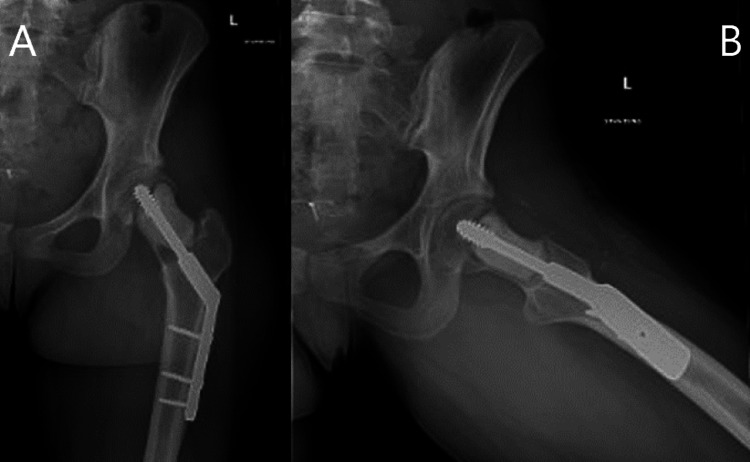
Postoperative radiographic anteroposterior (A) and frog-leg lateral (B) views of the left hip after curettage and PMMA placement PMMA - polymethylmethacrylate
